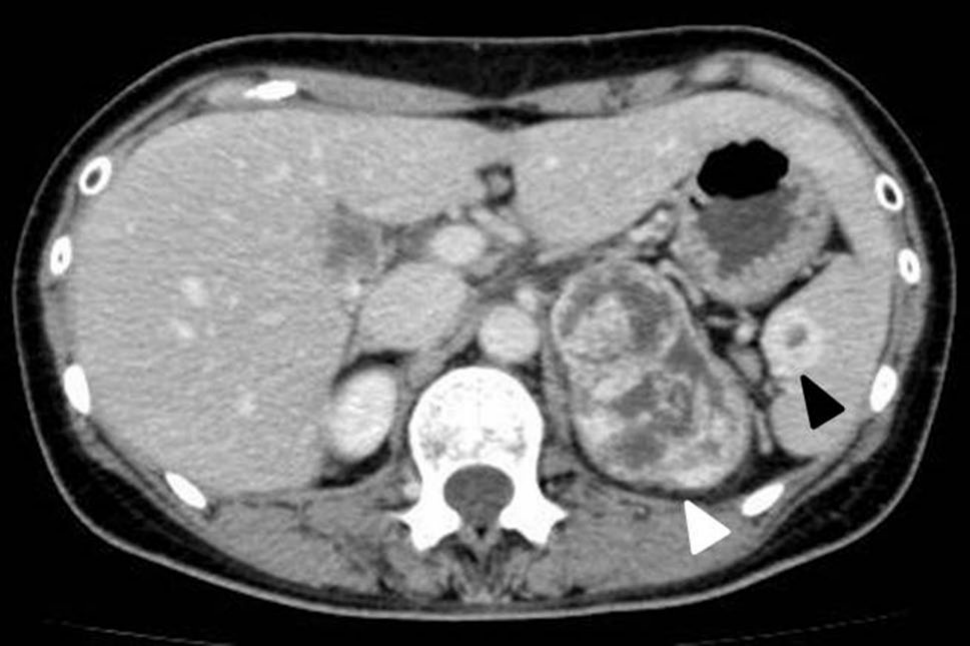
Computed tomography examination showing a heterogeneously enhancing left adrenal tumor with several septa (white allow) and an enhanced spleen mass (black allow)
